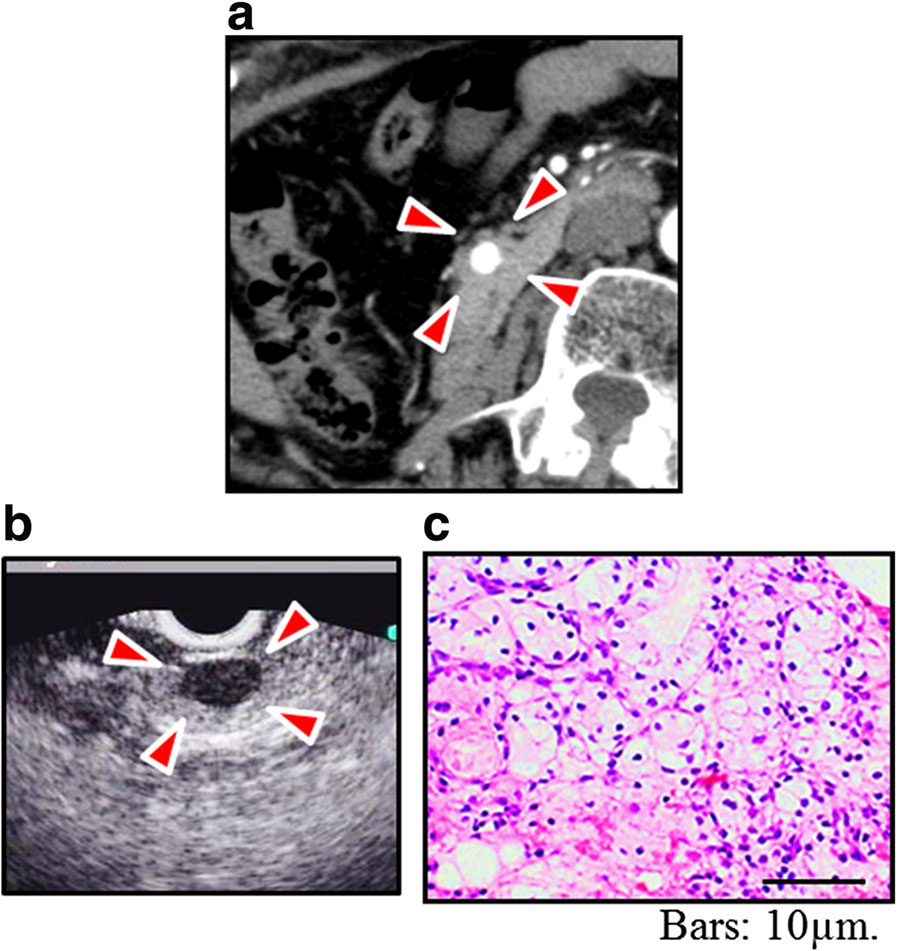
Preoperative findings of case no. 6. Enhanced CT (a) and EUS (b) revealed 10-mm tumor (*arrowheads*) in the pancreatic head of case no. 6. It was preoperatively diagnosed as metastasis from clear cell RCC (c) by EUS-FNA
